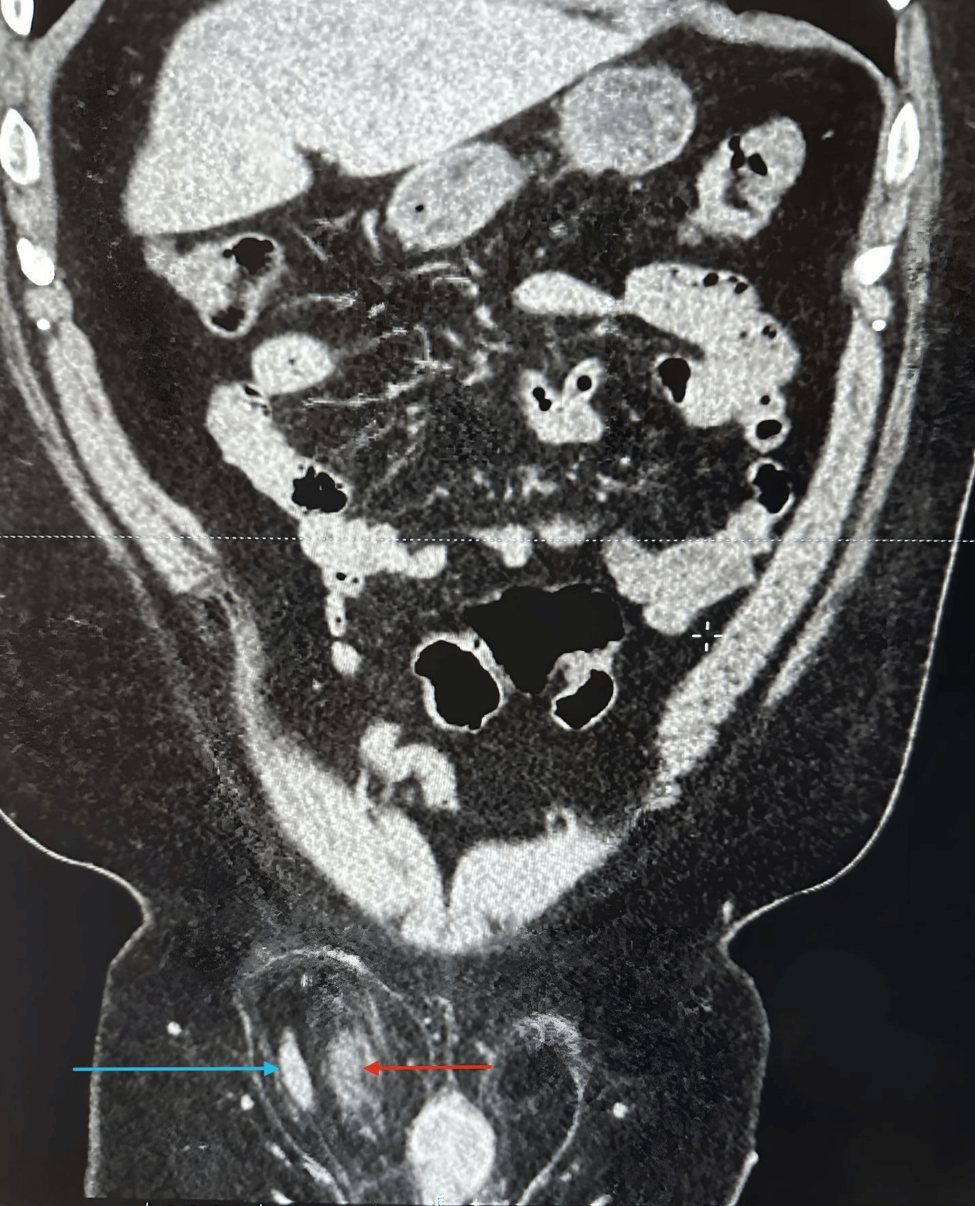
Coronal CT scan with presence of both Amyand's hernia and IBH Blue arrow indicates appendix; red arrow indicates bladder IBH: inguinal bladder hernia
